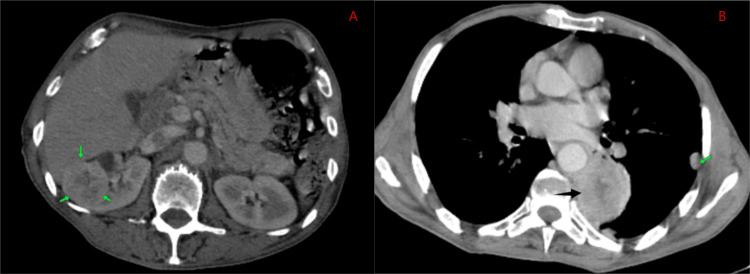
Thoraco-abdomino-pelvic CT scan. (A) Presence of a highly suspicious right renal mass (green arrow). (B) A mass in the dorsal segment of the culmen (black arrow), associated with a subpleural nodule (green arrow).
